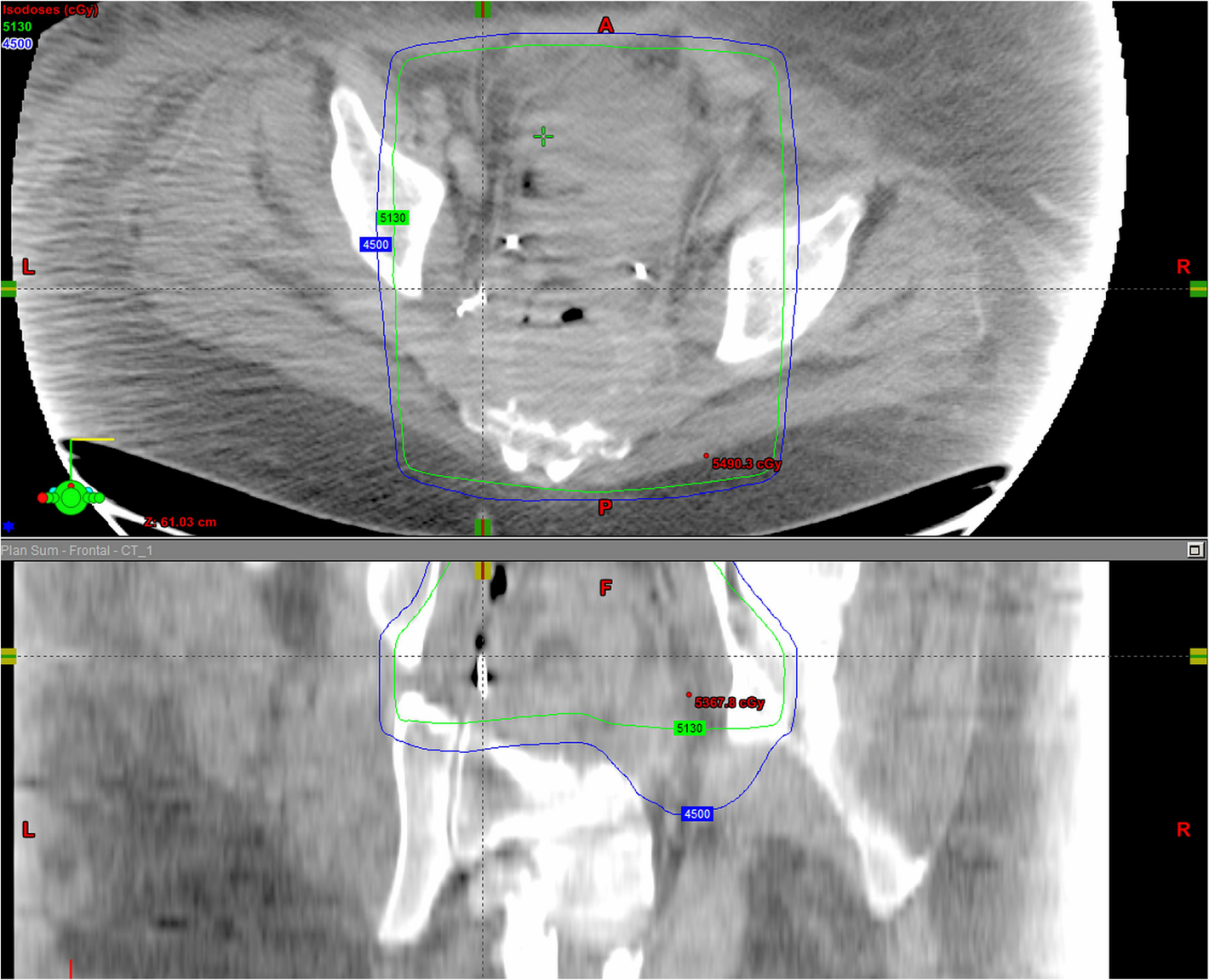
Radiation dose distribution images superimposed on CT angio abdomen and pelvis following PSA embolization. The PSA is noted to be within the 95% isodose line (5130 cGy, green line). Of note bilateral ureteral stents are seen anterior to the left pelvic embolization coils
